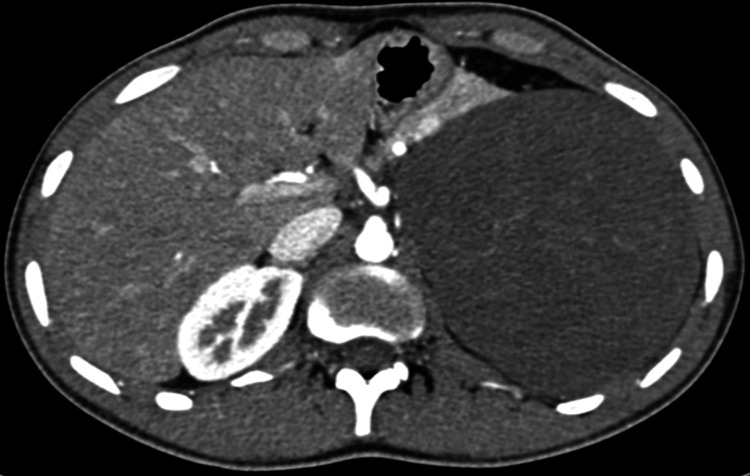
CT before surgical resection of the tumor. Large mass present in the left upper abdominal quadrant, causing anterior and superior displacement of the spleen, anterior displacement of the pancreas and stomach, and inferior displacement of the left kidney. It measures approximately 15.9 x 11.8 x 13.5 cm. The left adrenal gland is not visualized. The mass appears to be separable from the spleen, pancreas and left kidney. The mass shows internal vascular structures in the arterial phase with an average density of 44 HU in the porto-venous phase.
